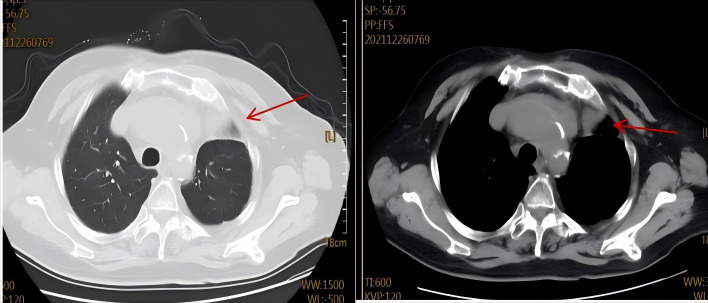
Treatment response assessment after first-line chemotherapy. Follow-up chest CT scan shows a significant reduction in the size of the anterior mediastinal mass (red arrow), consistent with a partial response.
